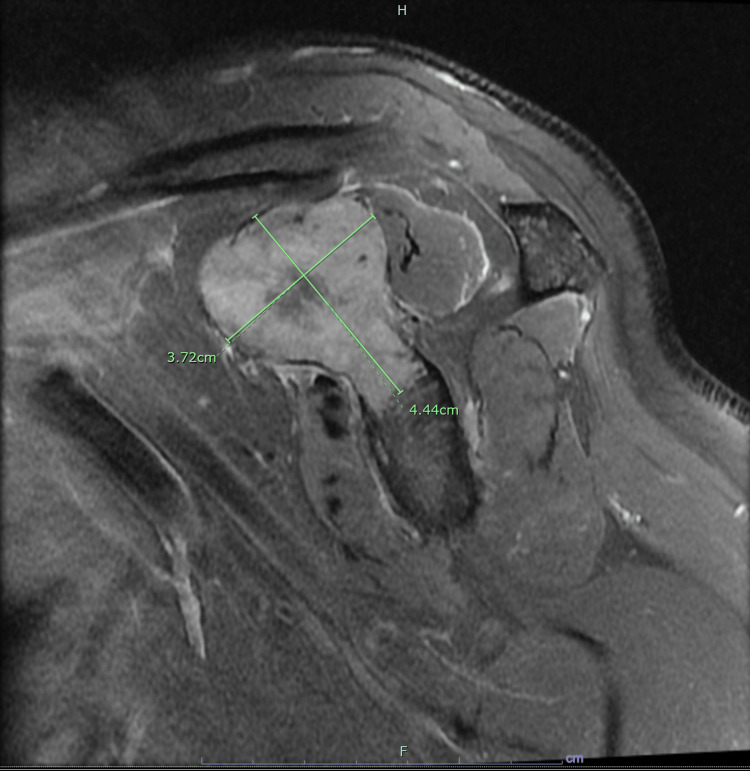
MRI without contrast of the left shoulder, left scapula Multiple metastatic masses are seen among the shoulder with the largest in the scapula at the level of the coracoid process measuring 3.7 x 4.4 cm MRI: magnetic resonance imaging
